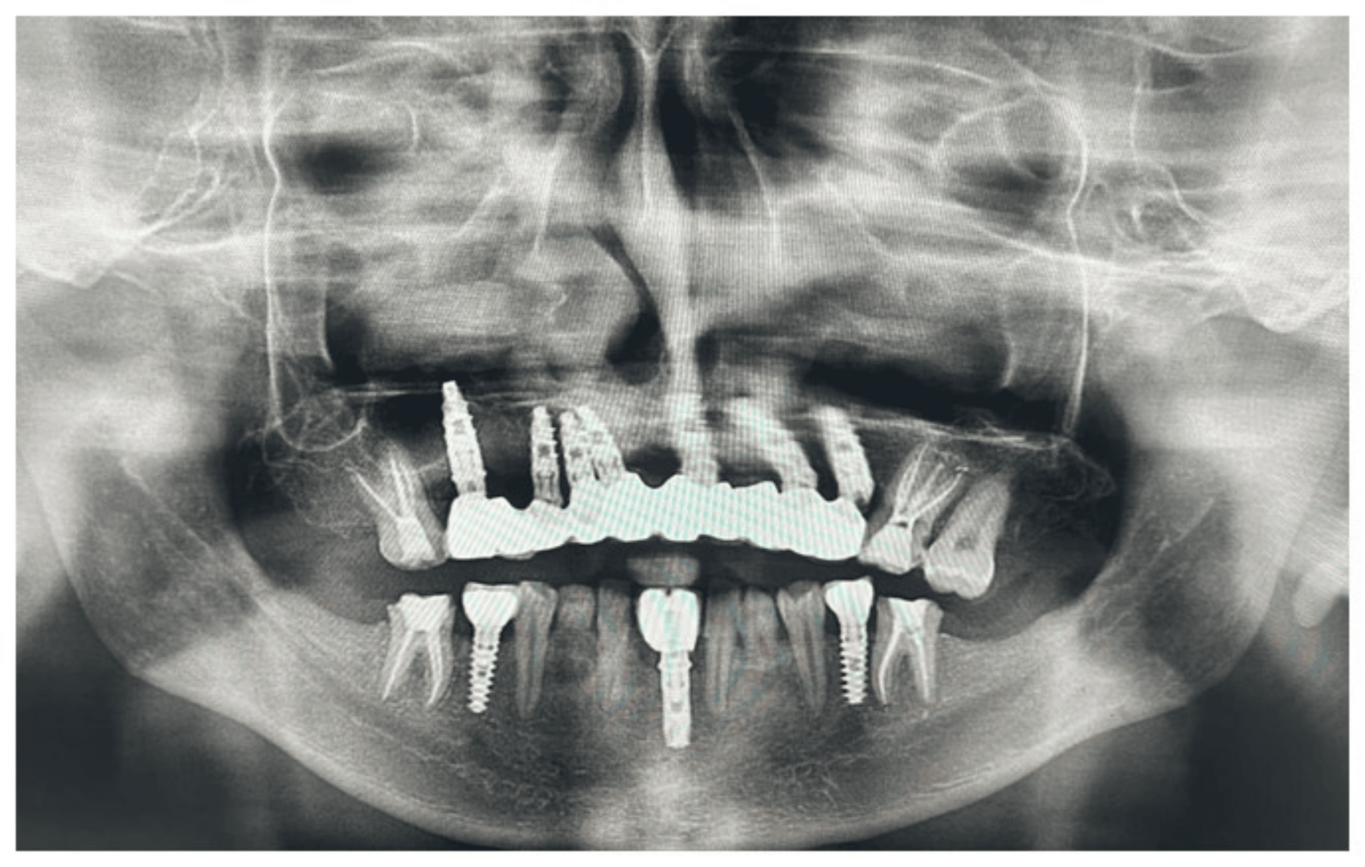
Preoperative radiograph (OPG) OPG: orthopantomography
